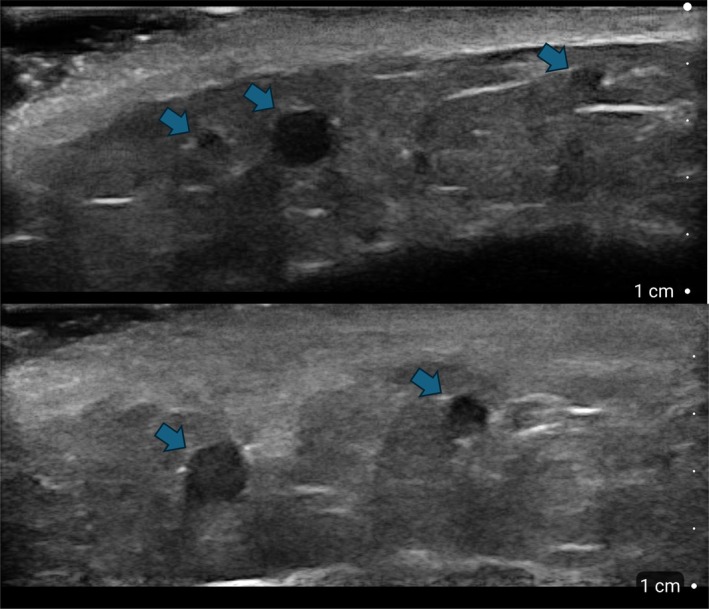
High‐frequency ultrasonography (HFUS) findings. Representative 20‐MHz HFUS image showing well‐defined, avascular, and hypoechoic nodules (blue arrows) at the dermal–subcutaneous interface, highly suggestive of foreign‐body granulomas.
